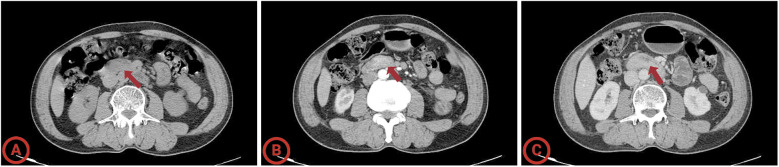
Computed tomography of the giant sporadic non-ampullary duodenal adenoma. (A) Arterial phase; (B) venous phase; (C) delayed scan. The average HU values of the lesion in the plain scan, arterial, and portal venous phases are 63.2 HU, 87.9 HU, and 93.1 HU.
